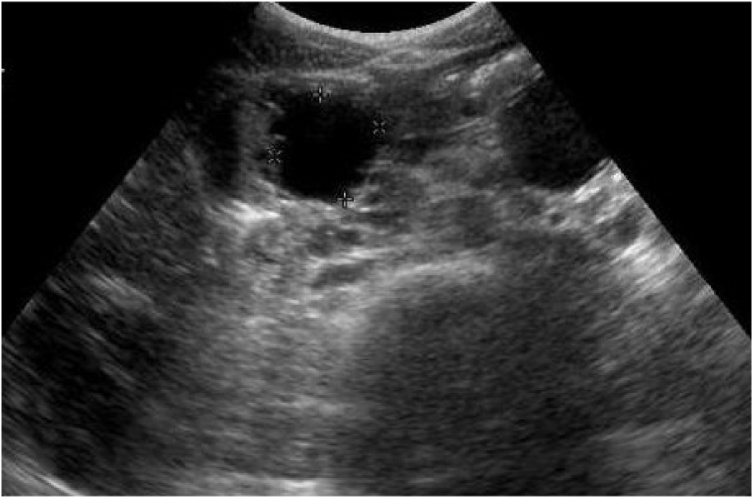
Ultrasonogram of abdomen showing well defined hypoechoic mass arising from body of pancreas.
